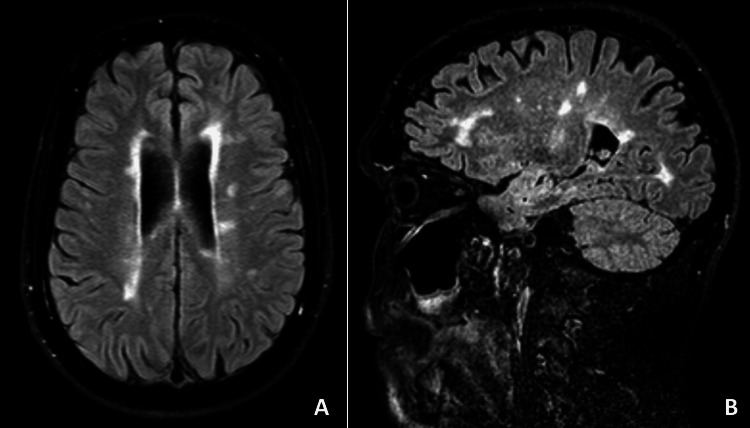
Brain magnetic resonance imaging These images show multiple infracentimetric lesions with hyperintense signals in fluid-attenuated inversion recovery (FLAIR) images, with evidence of space (multiple lesions in different locations) and time (lesions with different contrasts uptake) dissemination, suggestive of primary demyelinating disease: (A) axial (transverse) plane; (B) sagittal plane.
